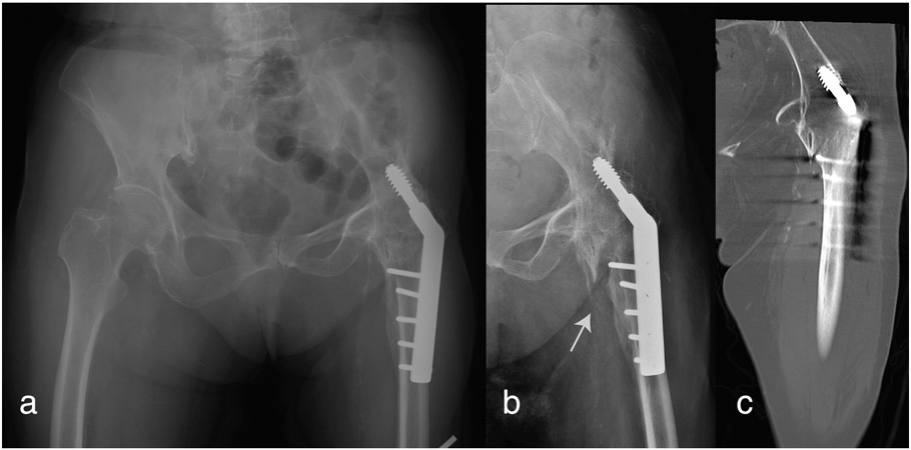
a) Pre-injury radiograph of an 88-year-old lady showing solid arthrodesis of the left hip with a DHS. b) X-ray showing an intertrochanteric fracture after injury. c) Reformatted coronal CT scan revealing the fracture in the trochanteric region with suspected loosening of the DHS lag screw.
